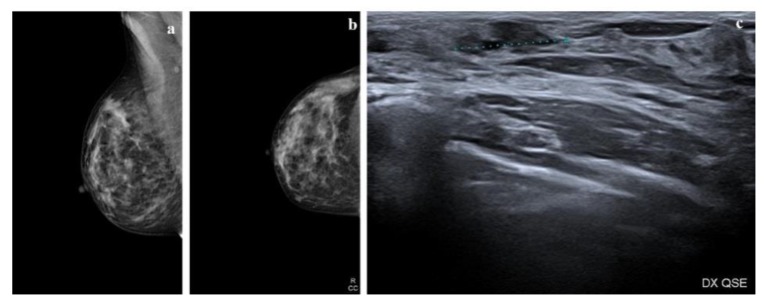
Radiologic features of a low-grade ductal carcinoma in situ (DCIS) lesion. (a,b) Mammograms show a heterogeneously dense right breast. In the upper outer quadrant (UOQ) there is a small oval opacity with obscured margins; (c) ultrasound shows an irregular mass with indistinct margins, hypoechoic echo pattern without posterior features.
